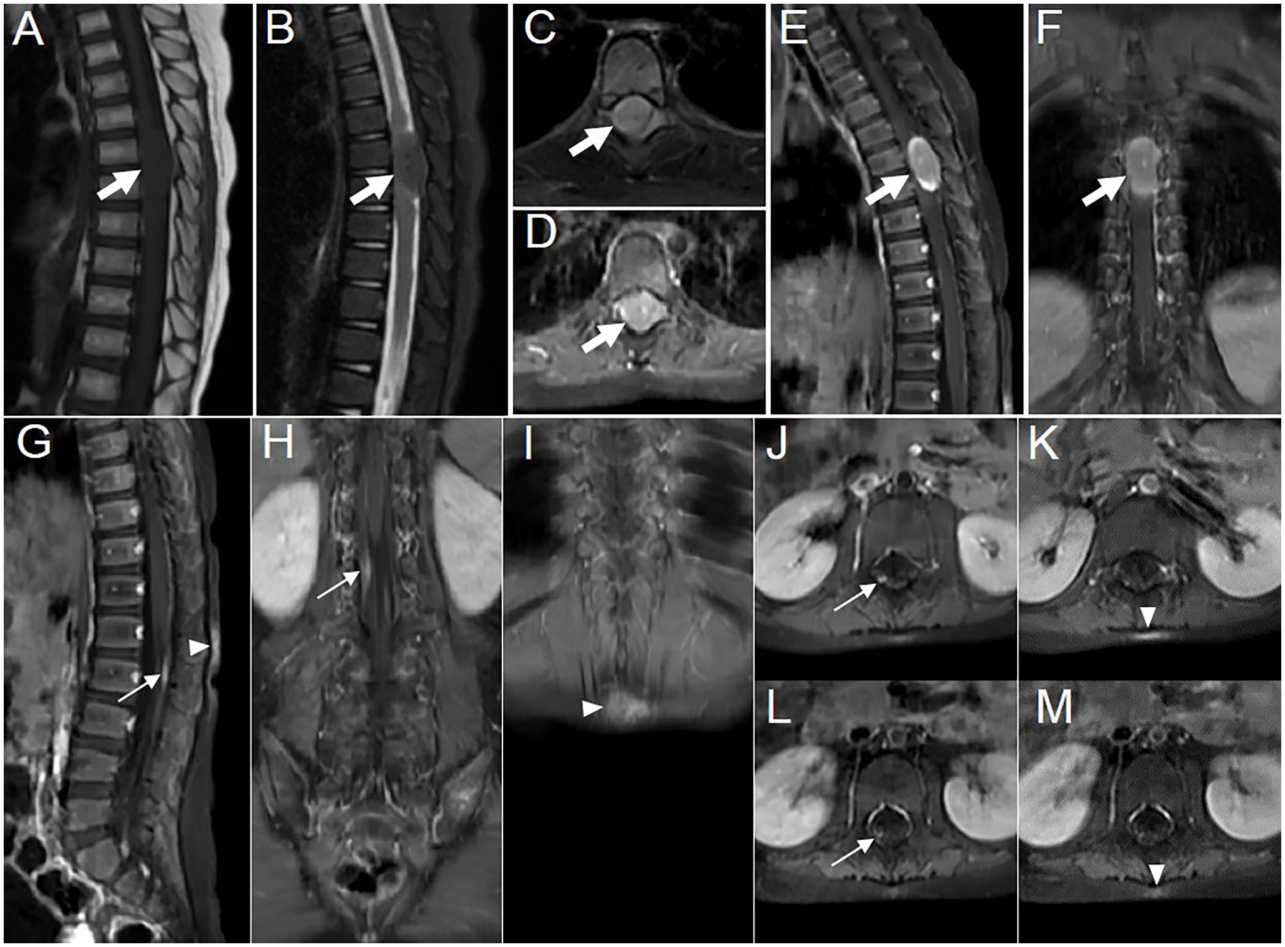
Images showing multisystem lesions in the 3-year-old girl with APH. (A–C) MR images showing hypointense on sagittal T1WI (A, thick arrow) and isointense with central punctate hypointense areas on sagittal and axial fat-suppressed sequences of T2WI (B, C, thick arrow). (D–F) Contrast-enhanced T1WI revealing moderate enhancement of the intradural extramedullary nodule, with marked enhancement at the upper and lower rims, accompanied by markedly punctate enhancement in the center on axial (D, thick arrow), sagittal (E, thick arrow), and coronal (F, thick arrow) scans. (G–I) Contrast-enhanced T1WI showing segmentally moderate enhancement of the lesions in the leptomeninges (thin arrow) and the dorsal skin at the L1–2 level (arrowhead) on sagittal (G) and coronal (H, I) scans. Axial contrast-enhanced T1WI showing the changes in leptomeninges and skin lesions before (J, K) and after (L, M) treatment, respectively.
